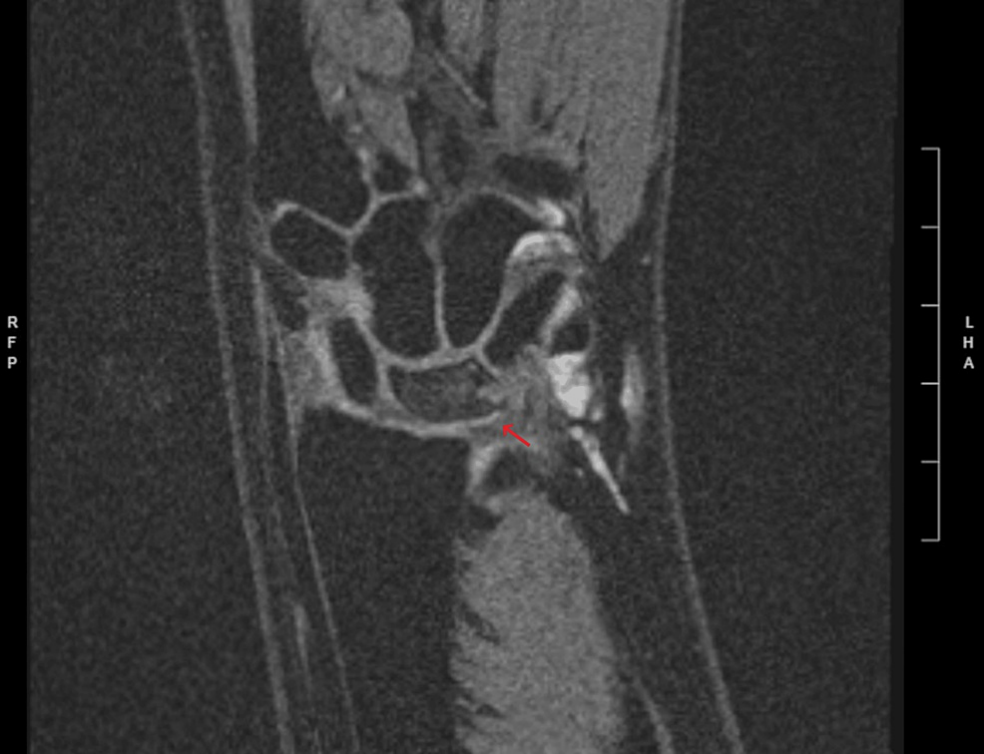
Post-treatment T2 coronal MRI The arrow corresponds to diffuse increased signal intensity, which is similar to prior T2 coronal MRI. Image #10/19; spin echo (SE): 4001
